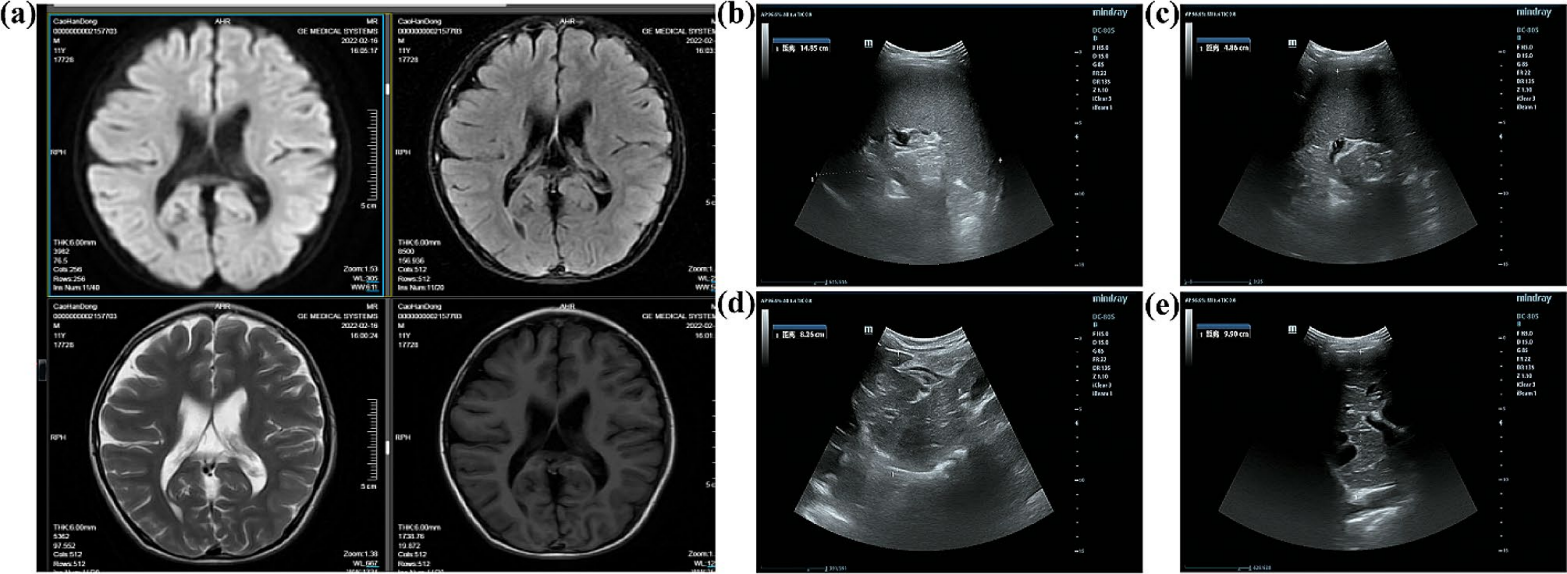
Brain CT and abdominal ultrasound of the patient. (a) A CT scan of the patient’s brain showed a slight widening of the sulcal fissure. (b, c) Length and thickness of the patient’s spleen. (d, e) Anteroposterior diameter of the left and right lobes of the liver
